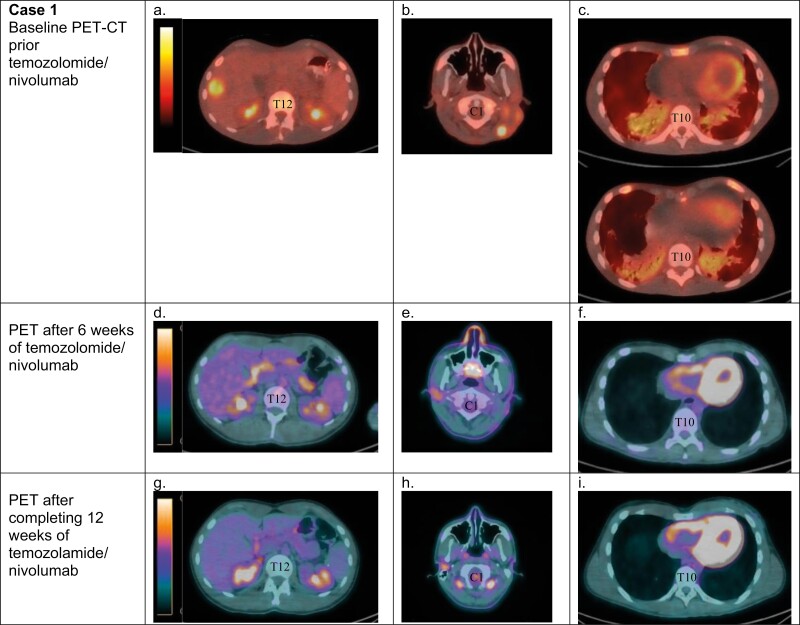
Case 1, 19-year-old male with metastatic melanoma. Baseline PET-CT prior to temozolomide/nivolumab showed new FDG avid segment 5/6 hepatic metastasis (a), increased FDG avid left neck subcutaneous and cutaneous metastases (b), and new FDG avid bilateral lower lobe consolidations (c). After 6 weeks of temozolomide/nivolumab, the liver metastasis decreased in size and no longer demonstrated uptake above background (d), the cutaneous/subcutaneous lesions in the left neck decreased in size and intensity of uptake with only mild residual uptake (e), and there was interval resolution of bilateral lower lobe consolidations (f). After 12 weeks of temozolomide/nivolumab, there were no suspicious FDG avid lesions in the abdomen or pelvis (g), no FDG avid cervical lymph nodes (h), and no FDG avid or otherwise suspicious pulmonary nodules (i).
